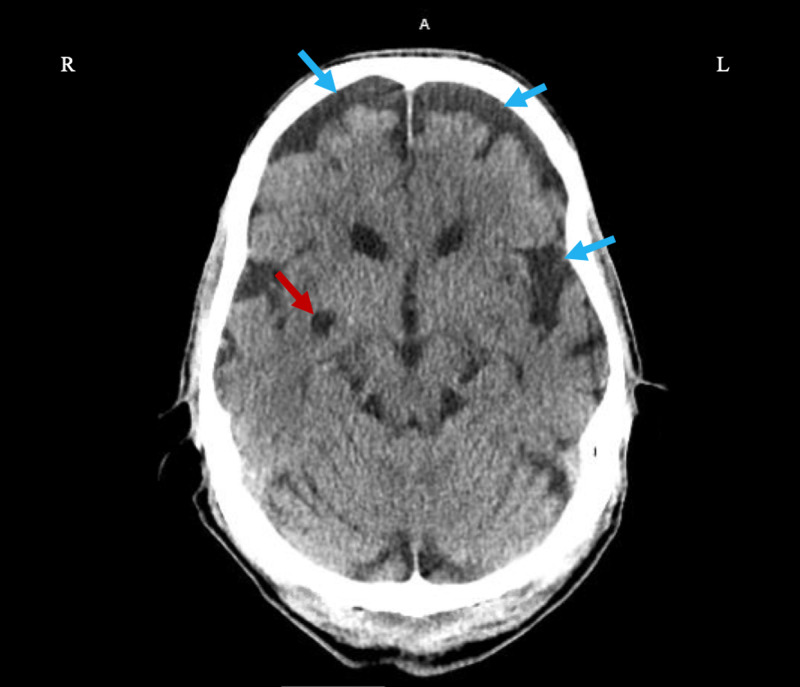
Axial Non-Contrast Head CT Diffuse brain atrophy (blue arrows) and old left lacunar infarct (red arrow).
